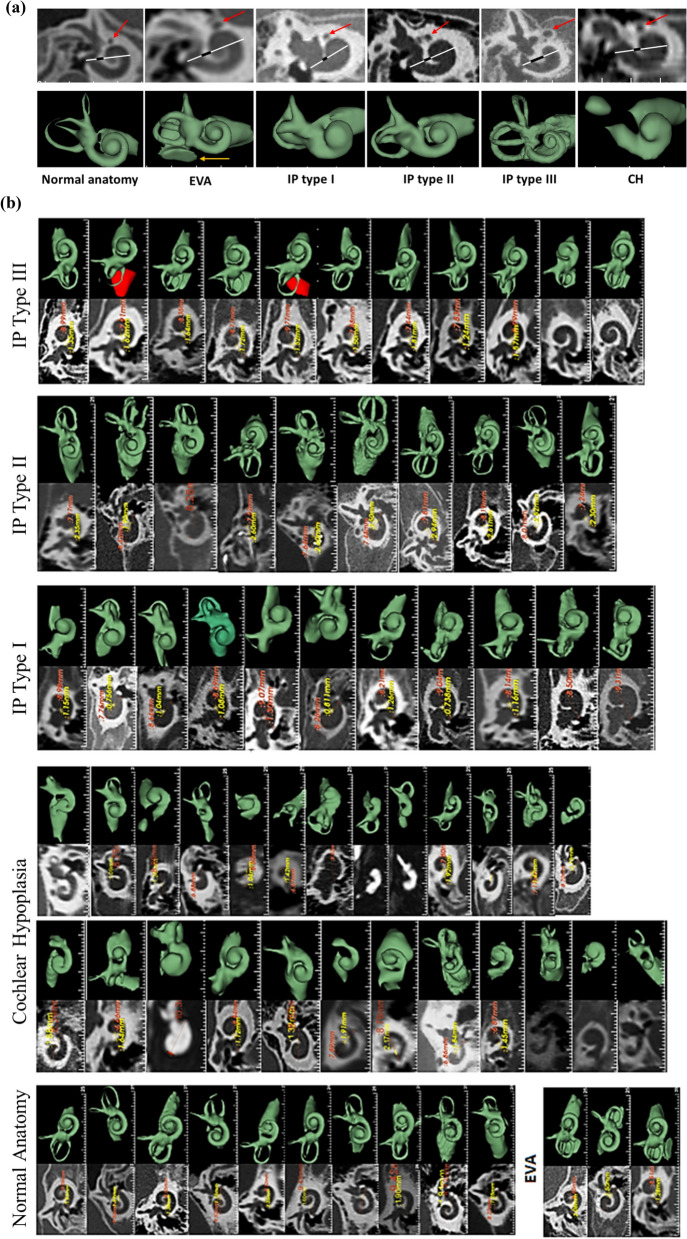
Two- and three-dimensional images of different cochlear anatomies, displayed in the oblique coronal plane/cochlear view, showing the A-value (white line), C-value (black line) and the facial nerve (red arrow) (a); and all seventy-four HRCT images of the cochlear samples used in the analyses along with the corresponding 3D images (b).
